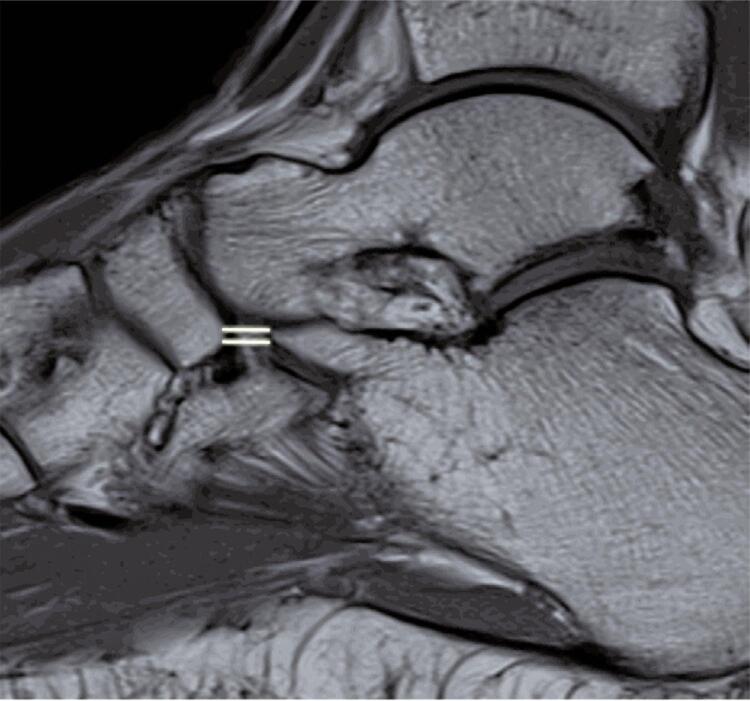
Calcaneonavicular distance was measured using a sagittal T1-weighted image that includes the tip of the anterior calcaneal process and the proximal lateral pole of the navicular (double line)
